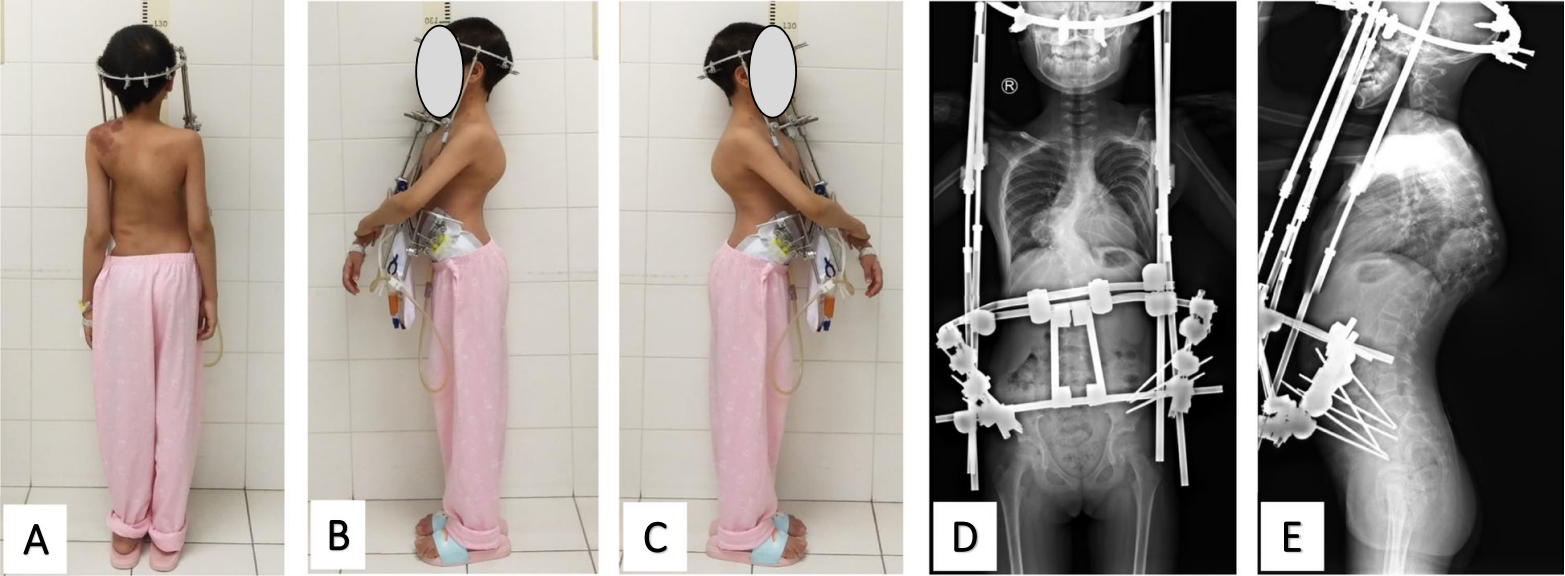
The halo-pelvic traction. The posterior (a) and lateral (b/c) views photographs, and the standing anteroposterior (d) and lateral digital radiographs (e)
